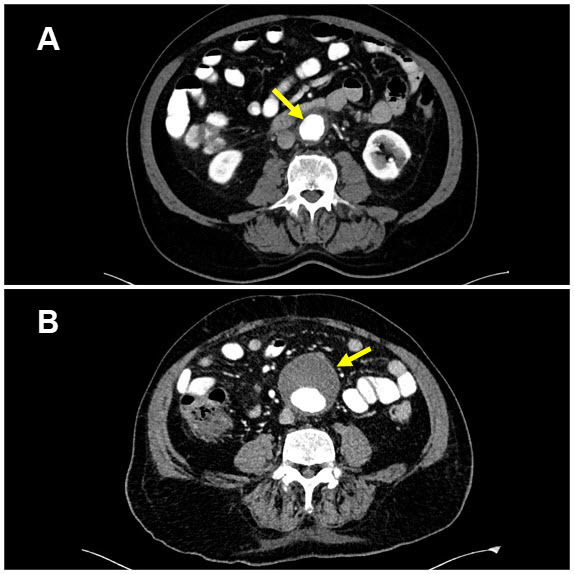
Color Doppler Ultrasound (CTA) Images of a Giant Cell Arteritis Patient. (A) A 2017 CTA showing active vasculitis of the abdominal aorta with aortic wall thickening (arrow); patient age 68 years. (B) The same patient in 2021 showing an aortic aneurysm (arrow); patient age 72 years.
